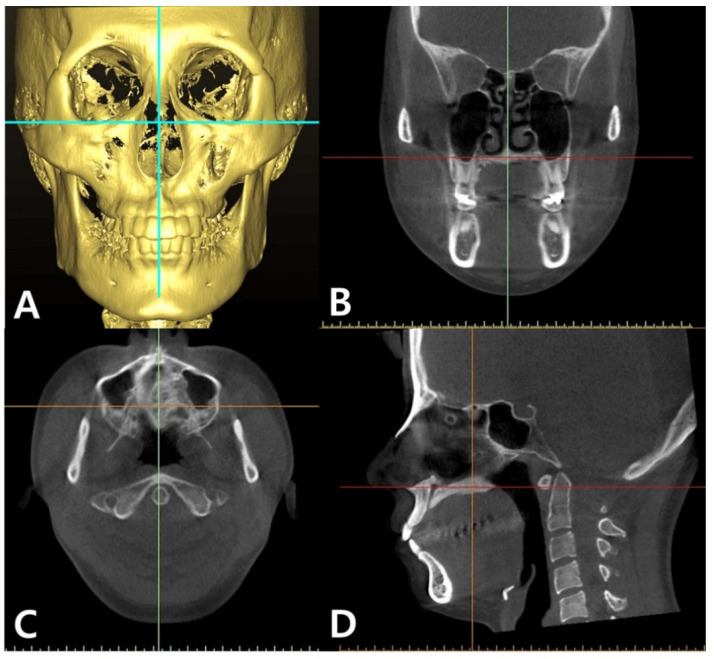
Process of cone-beam computed tomography (CBCT) image reorientation in the multiplanar orientation screen: (A) CBCT image was reorientated along the inferior border of the orbital rims in the frontal reconstructed view. (B,C) In the coronal and axial views, CBCT images were reoriented to allow for analysis at the midpalatal suture so that the green vertical line was positioned through the anterior nasal spine (ANS) to posterior nasal spine (PNS). (D) In the sagittal view, the CBCT image was rotated to confirm that the midpalatal line ran through ANS to PNS.
